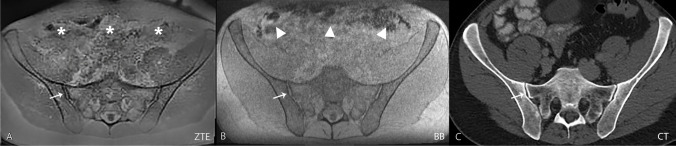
In this 42-year-old male patient referred for a kidney pathology, the SI joints were rated normal. Transverse images of ZTE (image A), BB (image B), and CT (image C) of the pelvis are shown without any pathologic findings in the SI joint. The cortical contour of the SI joint (thin arrows) is well-delineated in all three images. The ZTE image features a “flat soft tissue contrast” and thus emphasizes the bony contours of the pelvis. Gas in the bowel is more apparent in the black bone gradient echo (triangles) sequence compared to the ZTE sequence (asterisks). Abbreviations: SI = sacroiliac joint; ZTE = zero echo time; BB = black bone; CT = computed tomography
